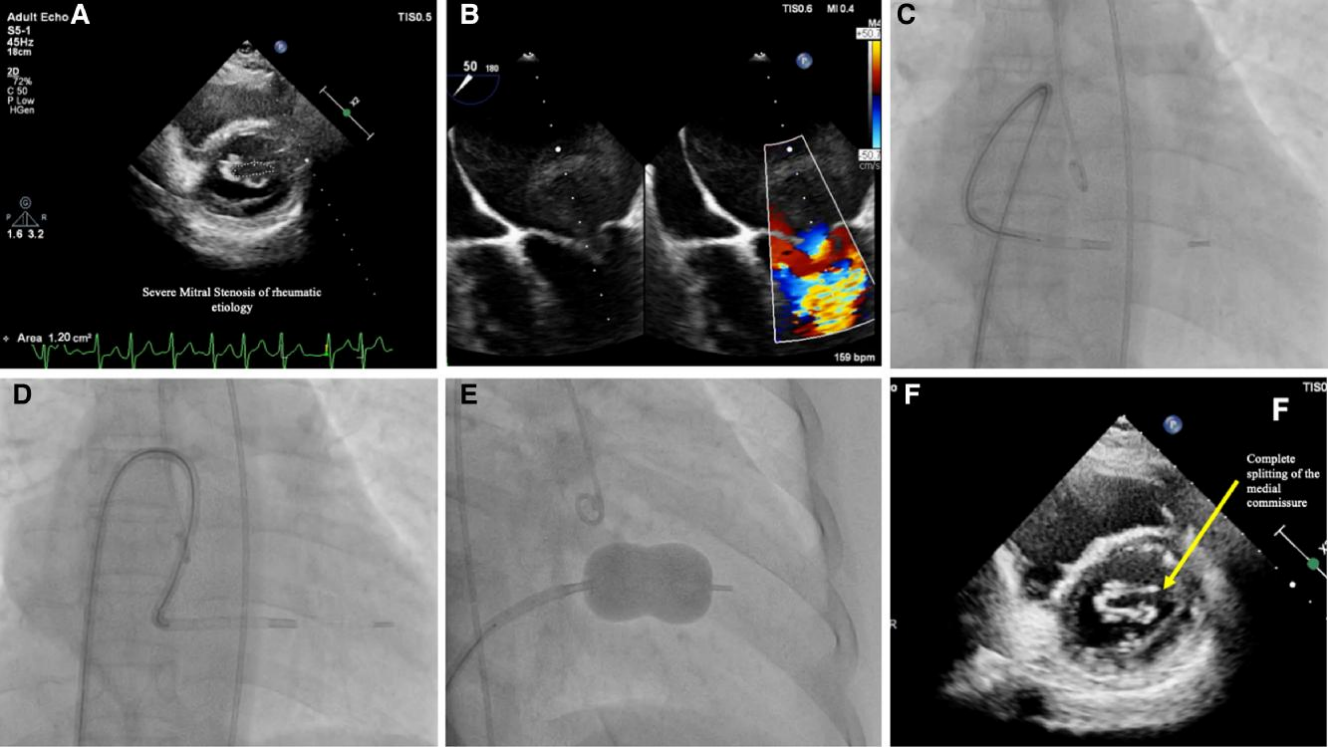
(*A*) Transthoracic echocardiography suggestive of severe mitral stenosis of rheumatic aetiology. (*B*) Transoesophageal echocardiography suggestive of mitral stenosis with hugely dilated left atrium. (*C*) Reverse (alpha) loop of balloon delivery catheter in left atrium. (*D*) Entry in the left ventricle across stenotic mitral valve. (*E*) Balloon inflation across mitral valve with relief of the lateral commissure. (*F*) Post-percutaneous balloon mitral valvuloplasty increase in mitral valve area with complete splitting of the medial commissure.
